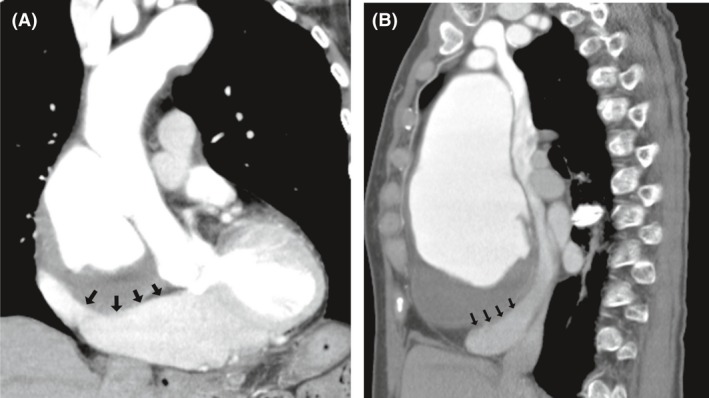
Preoperative contrast‐enhanced chest computed tomography. A (frontal view) and B (sagittal view) shows that a dilatation of the ascending aorta with dissection is observed. The right heart is stretched and distorted by the dilated false lumen (arrows).
